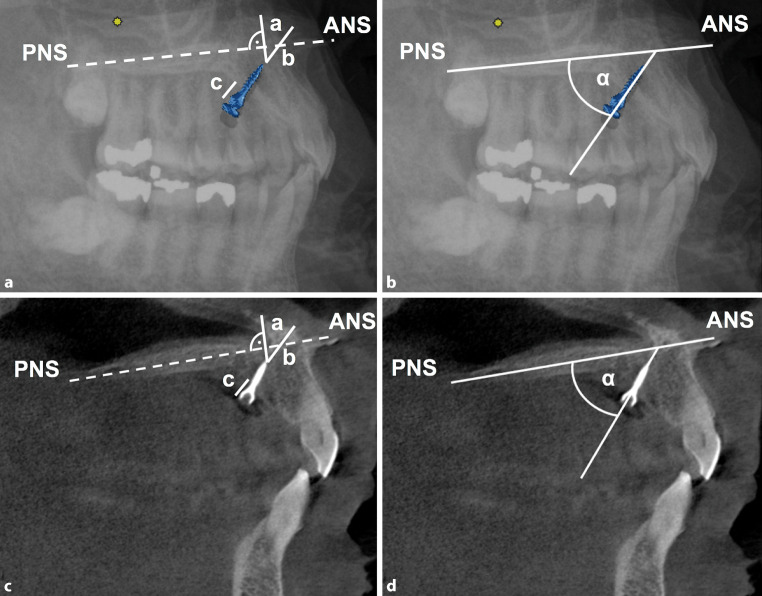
Radiographic measurement on lateral cephalogram (a, b) and the corresponding CBCT scans (c, d). *Line a* distance from the implant tip to the nasal floor at the right angle to the hard palate; *line b* straight distance from the implant tip to the nasal floor; *line c* posterior distance from the implant shoulder to the anterior hard palate and angle α: angle between the implant and palate plane (ANS-PNS) Radiologische Messungen in der Fernröntgenseitenaufnahme (a, b) und den korrespondierenden DVT (digitale Volumentomographie)-Aufnahmen (c, d). *Linie a* Abstand von der Implantatspitze zum Nasenboden im rechten Winkel zum harten Gaumen; *Linie b* gerader Abstand von der Implantatspitze zum Nasenboden; *Linie c* hinterer Abstand von der Implantatschulter zum harten Gaumen, Winkel α: Winkel zwischen Implantat und Gaumenebene (ANS-PNS)
